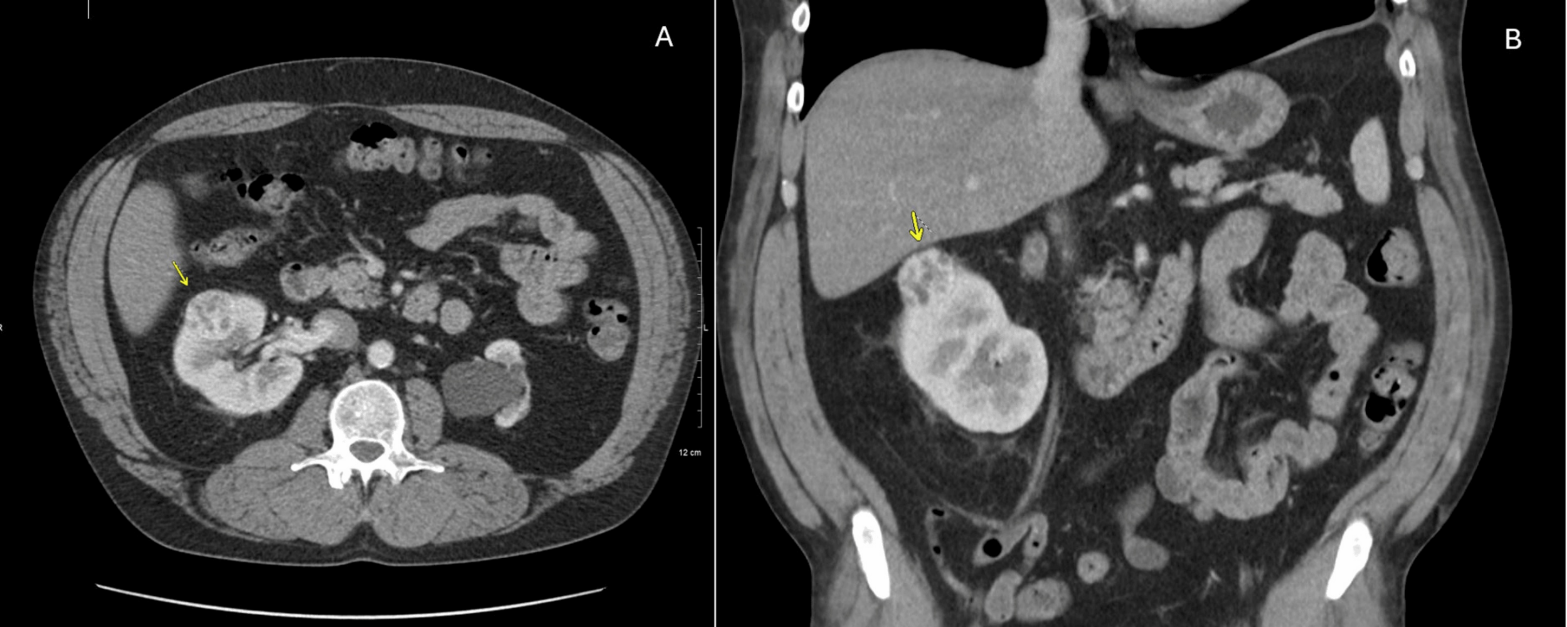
Axial, CT A/P w/ IV contrast (image A) and Coronal, CT A/P w/ IV contrast (image B) A 3.4 cm cortical mass is shown on axial (image A) and coronal (image B) views, with yellow arrows pointing to the mass. A/P: abdomen/pelvis
